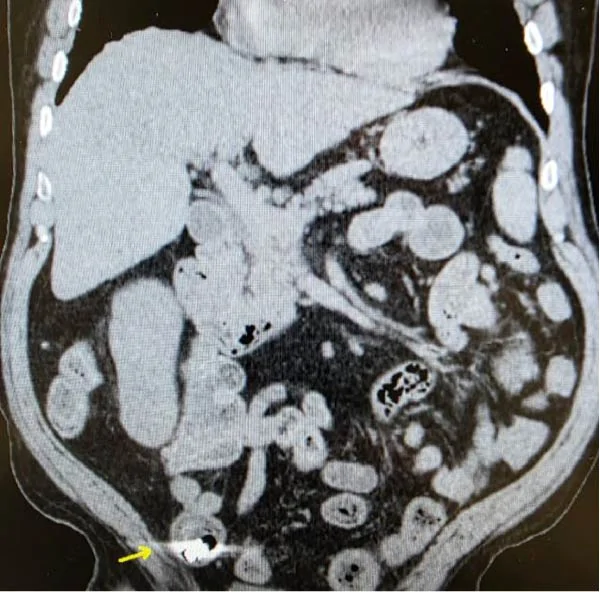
CT scan demonstrating the retained capsule.
